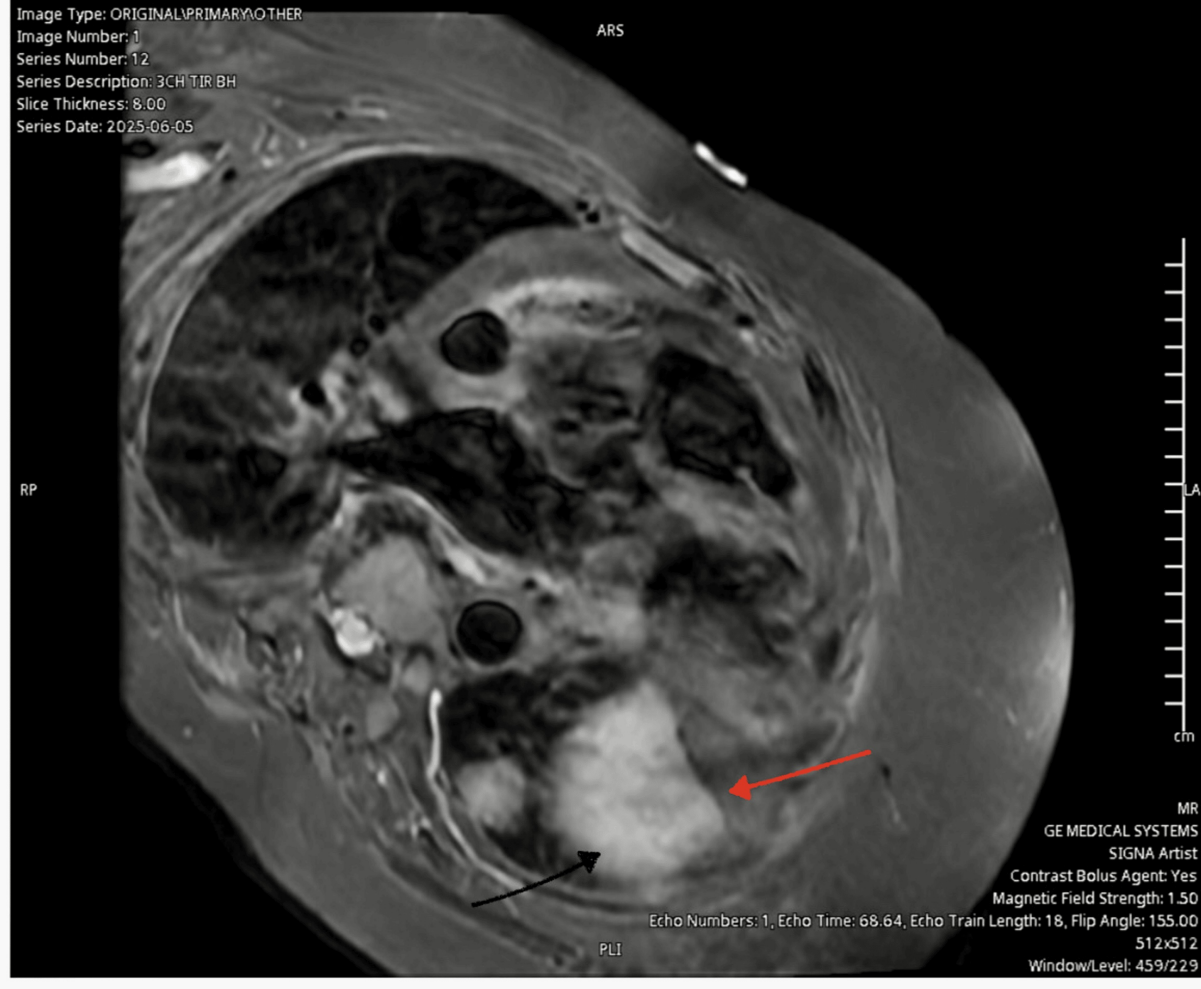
Cardiac MRI (3 chamber tau inversion recovery) showing increased signal intensities consistent with myocardial oedema and inflammation (red arrow)
